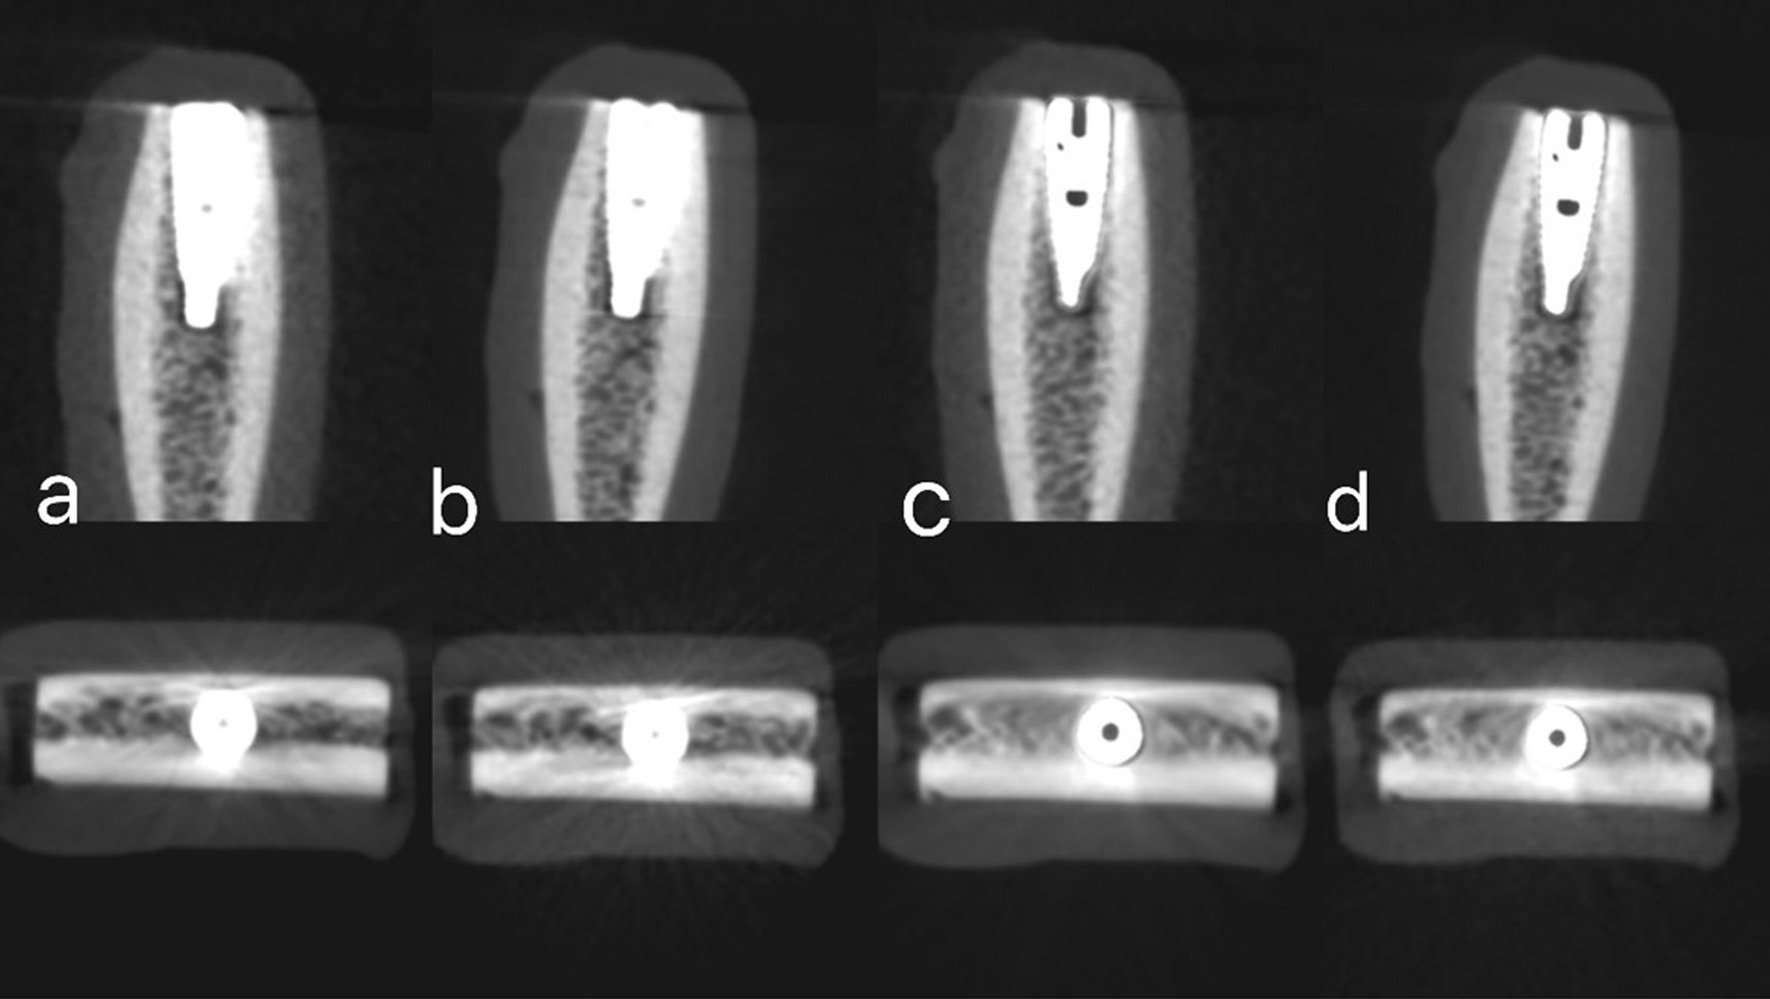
Sagittal and axial images with no peri-implant defect obtained with different four radiographic protocols. a High-dose CBCT without MAR. b Low-dose CBCT without MAR. c High-dose with MAR. d Low-dose CBCT with MAR
